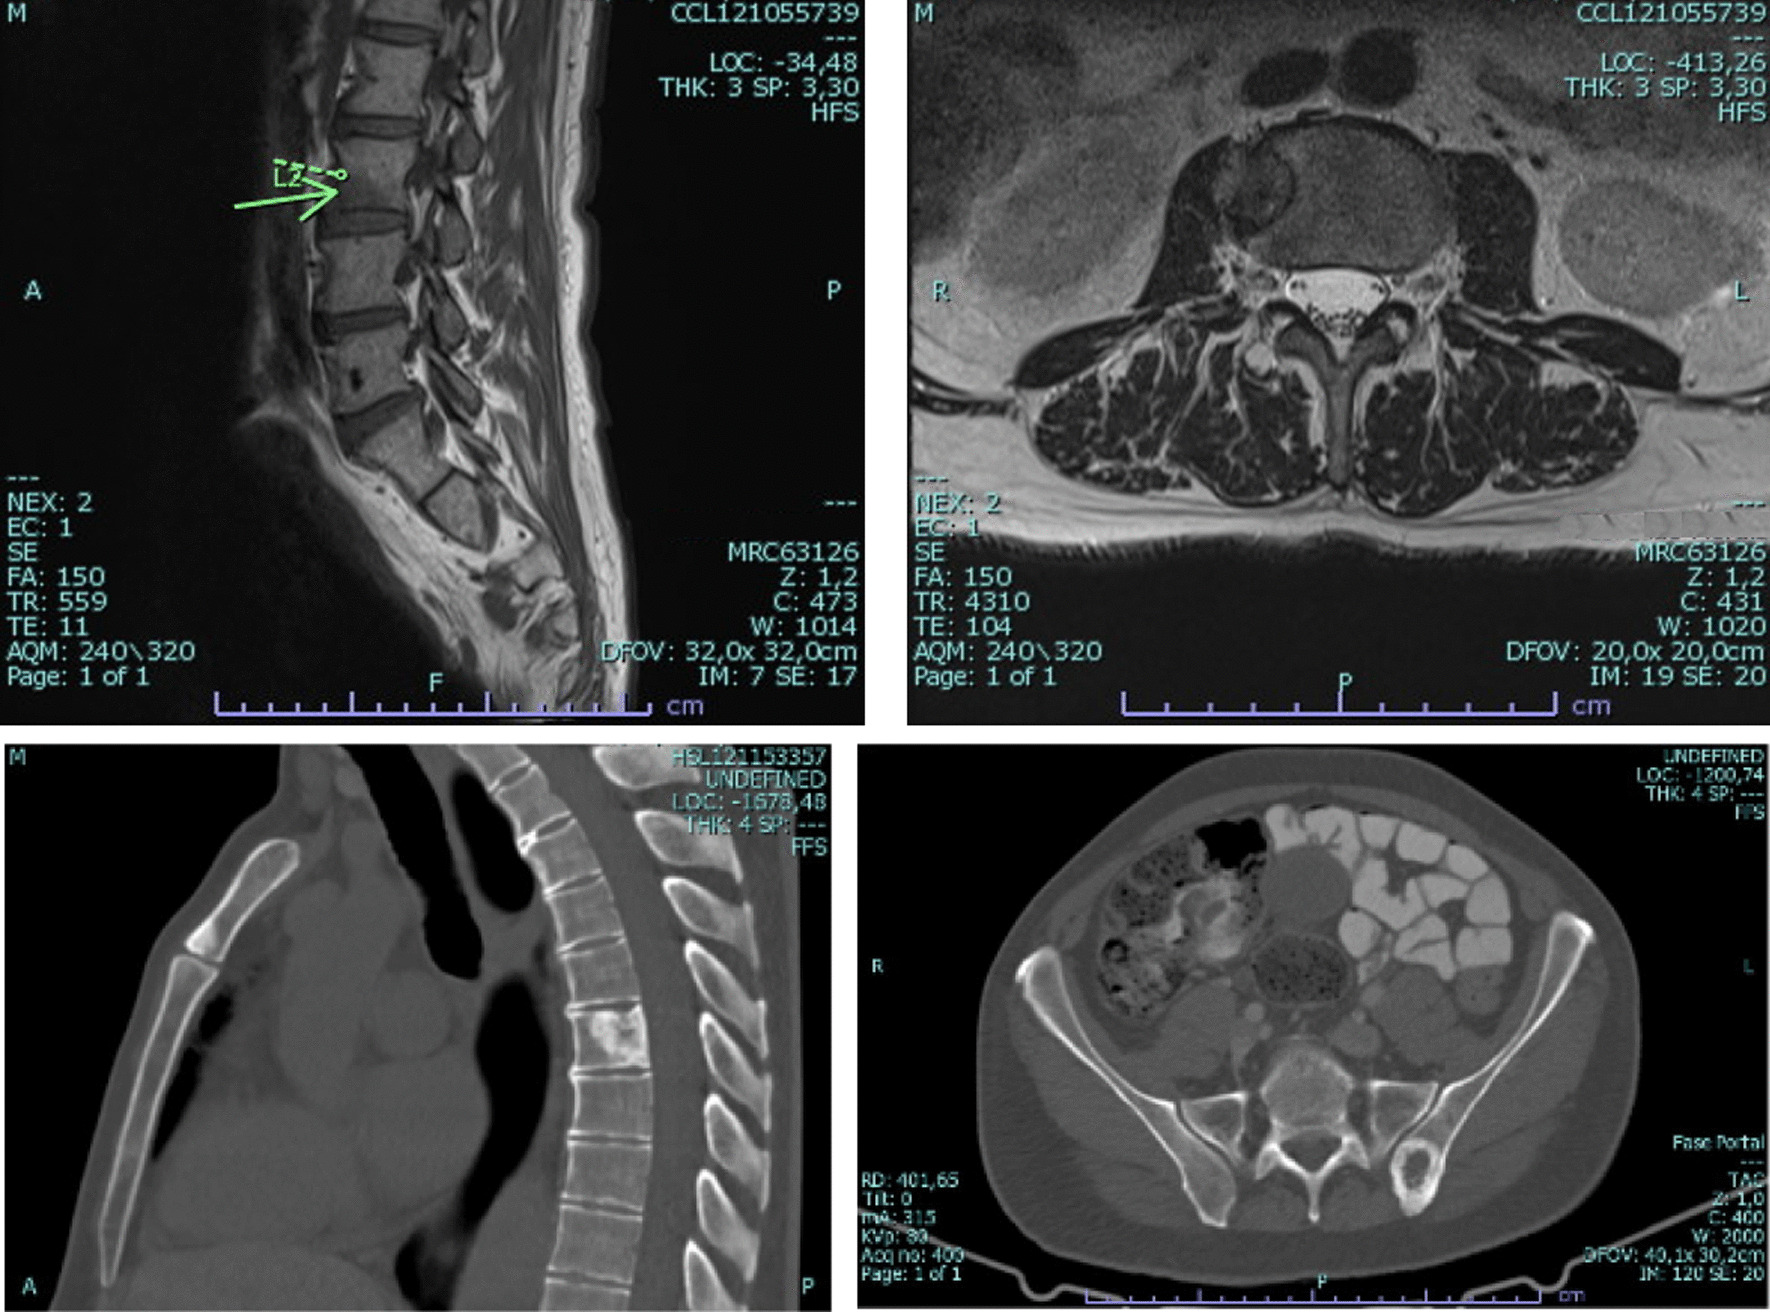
Computerized tomography and Magnetic resonance imaging of the cervical-dorsal-sacral spine revealing 4 metastatic bone lesions, again (on the arrow pointed in the image we see a de novo metastatic lesion in L2)–May 2021
